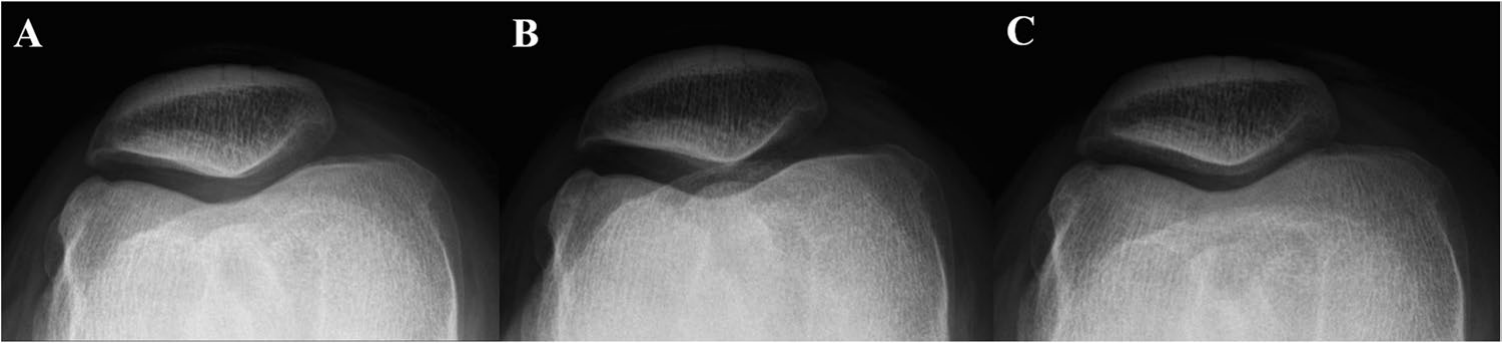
Examples of radiographs with beam projection angle deviations. A Neutral tilt, B 5° downward tilt, and C 5° upward tilt
